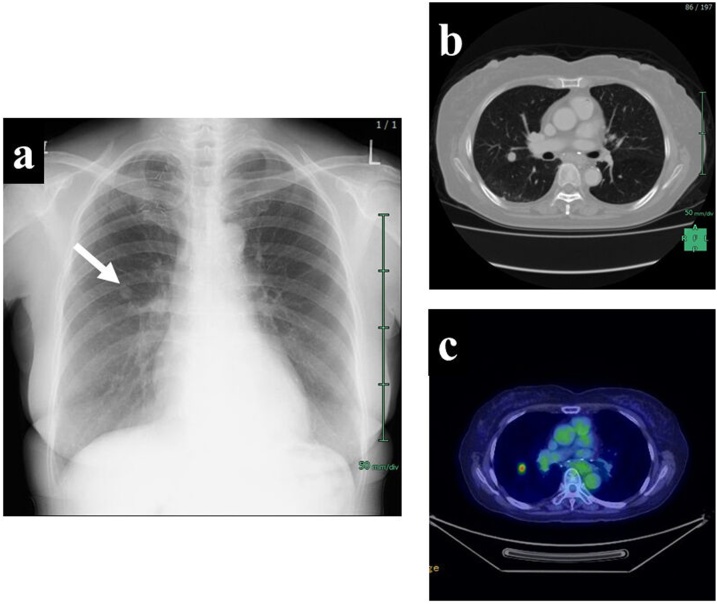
a: A chest X-ray at 7-year follow-up revealed a solitary pulomonary tumor at right lung (white arrow). b: A CT scan revealed the tumor with 1.5 cm of diameter. c: A PET-CT also showed the pulmonary tumor had abnormal uptake with 4.9 of standardized uptake value maximum.
